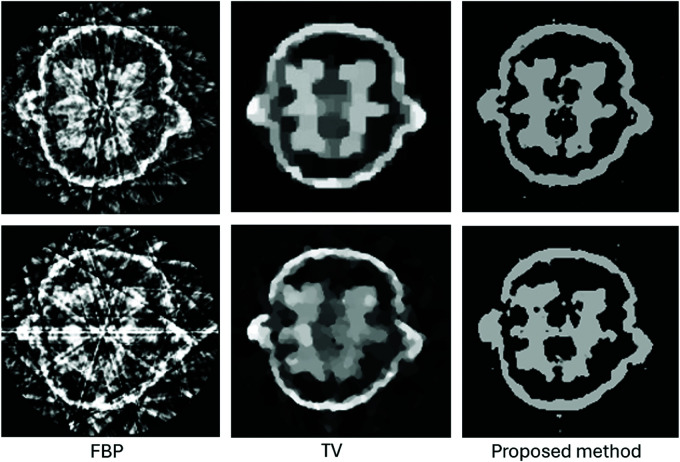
Comparison of reconstruction methods on real CT data of a walnut slice using 20 projections (top row) and 10 projections (bottom row). From left to right: Filtered Back Projection (FBP), Total Variation (TV) regularization, and the proposed method.
